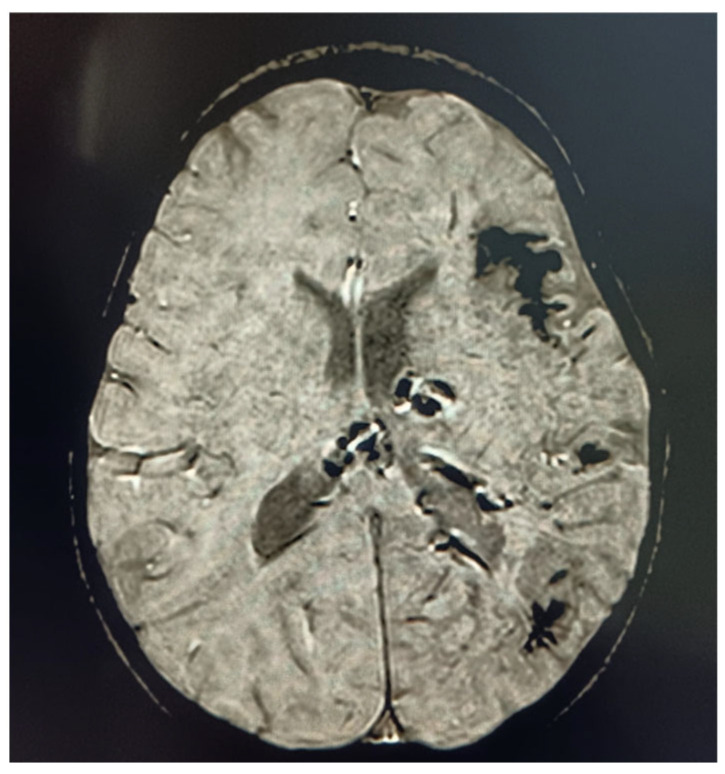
The MRI using the SWiP sequence reveals the characteristic radiological features of Sturge–Weber syndrome with multiple subcortical calcifications located in the fronto-insulo-temporal region but shows no evidence of recent ischemic areas.
